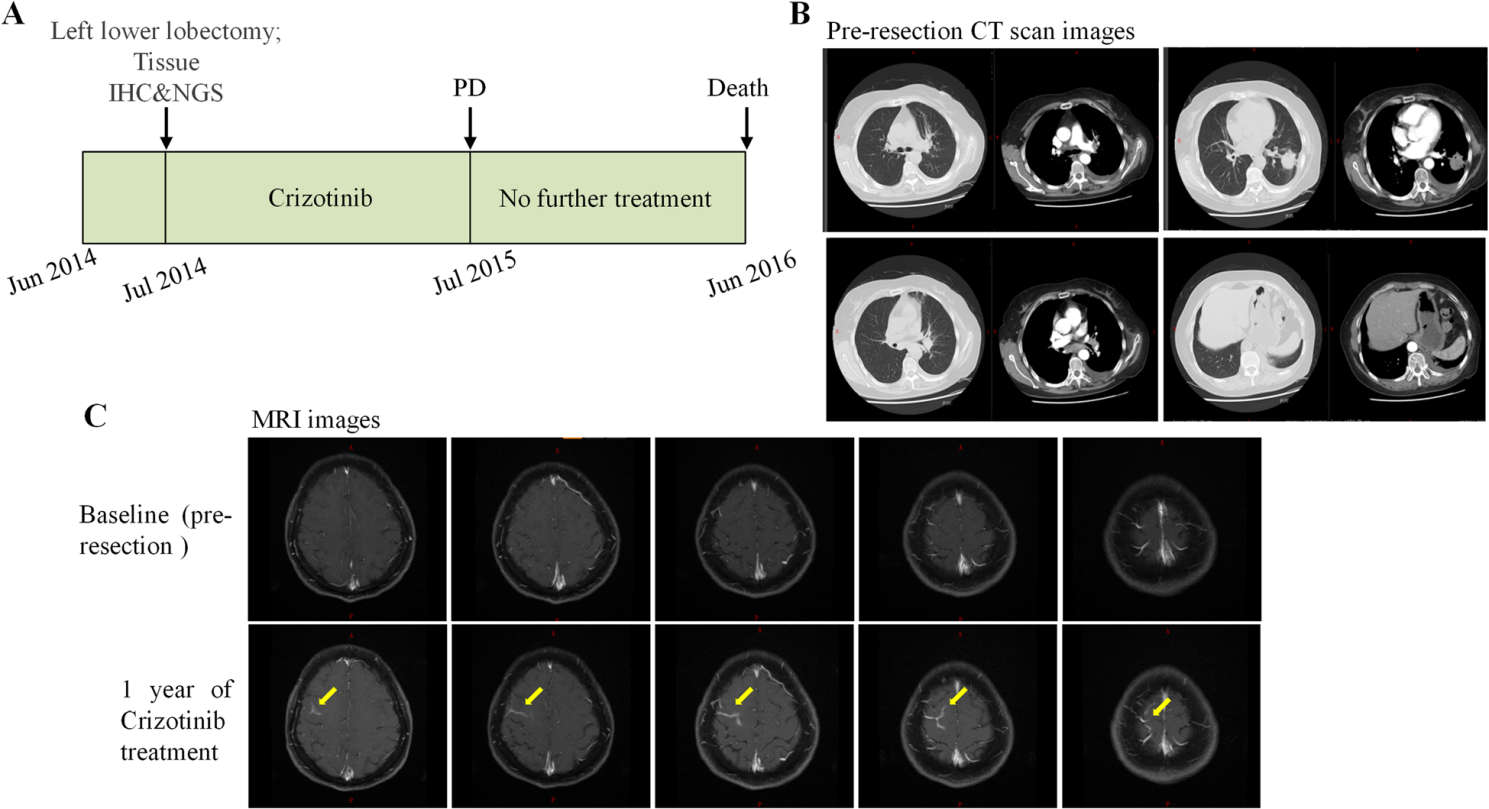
Timeline of the case 2’s disease from diagnosis to death (A). Chest computed tomography (CT) scans at diagnosis (B), and brain magnetic resonance imaging images at baseline (upper panel) and after crizotinib treatment (lower panel) (C). The yellow arrow indicates a new meningeal metastasis lesion
